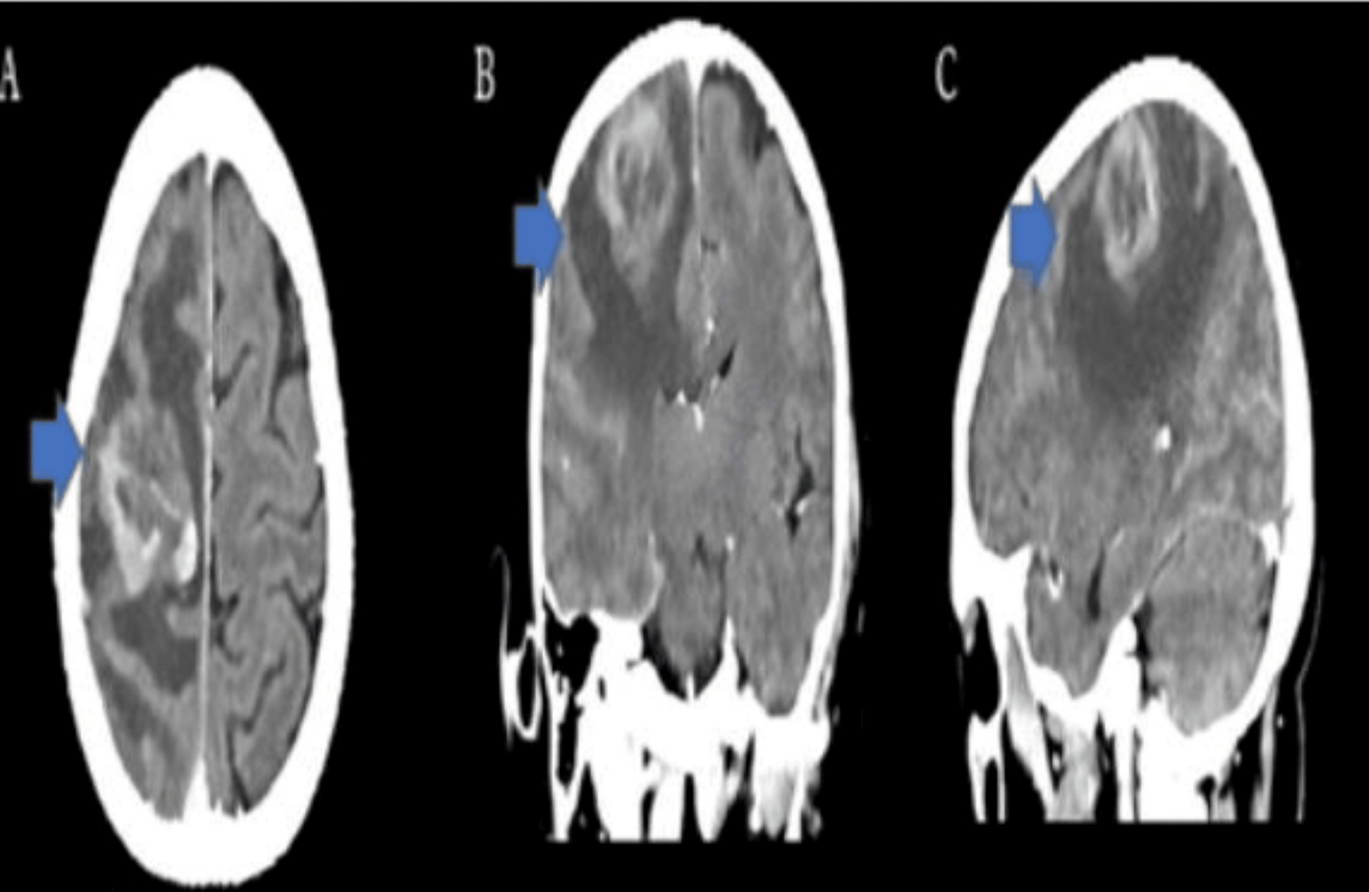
Axial (A), Coronal (B), and Sagittal (C) views of a contrast enhanced CT scan show an ill-defined space-occupying lesion measuring 3 x 3.9 x 3.4 cm in the right parietal lobe, surrounded by marked perifocal hypodense brain edema (highlighted by blue arrows)
